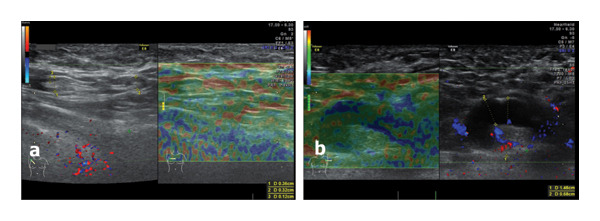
Ultrasound of the right axillary region of this patient 2 years postvaccination for COVID‐19 revealed normal lymph nodes with medium size of the transverse diameter and of the cortex, normal sinus, and low strain Score 2 Ueno (a). By comparison, in another patient, reactive lymph nodes after COVID‐19 vaccination demonstrated an early increase in transverse diameter size, thickening of the cortex with hypoechoic/transonic aspect, hypervasculature in sinus, and cortical BGR score suggesting edema (b). In the next 4–6 months after vaccination, we observed remission of inflammatory changes in the lymph nodes in all patients.
